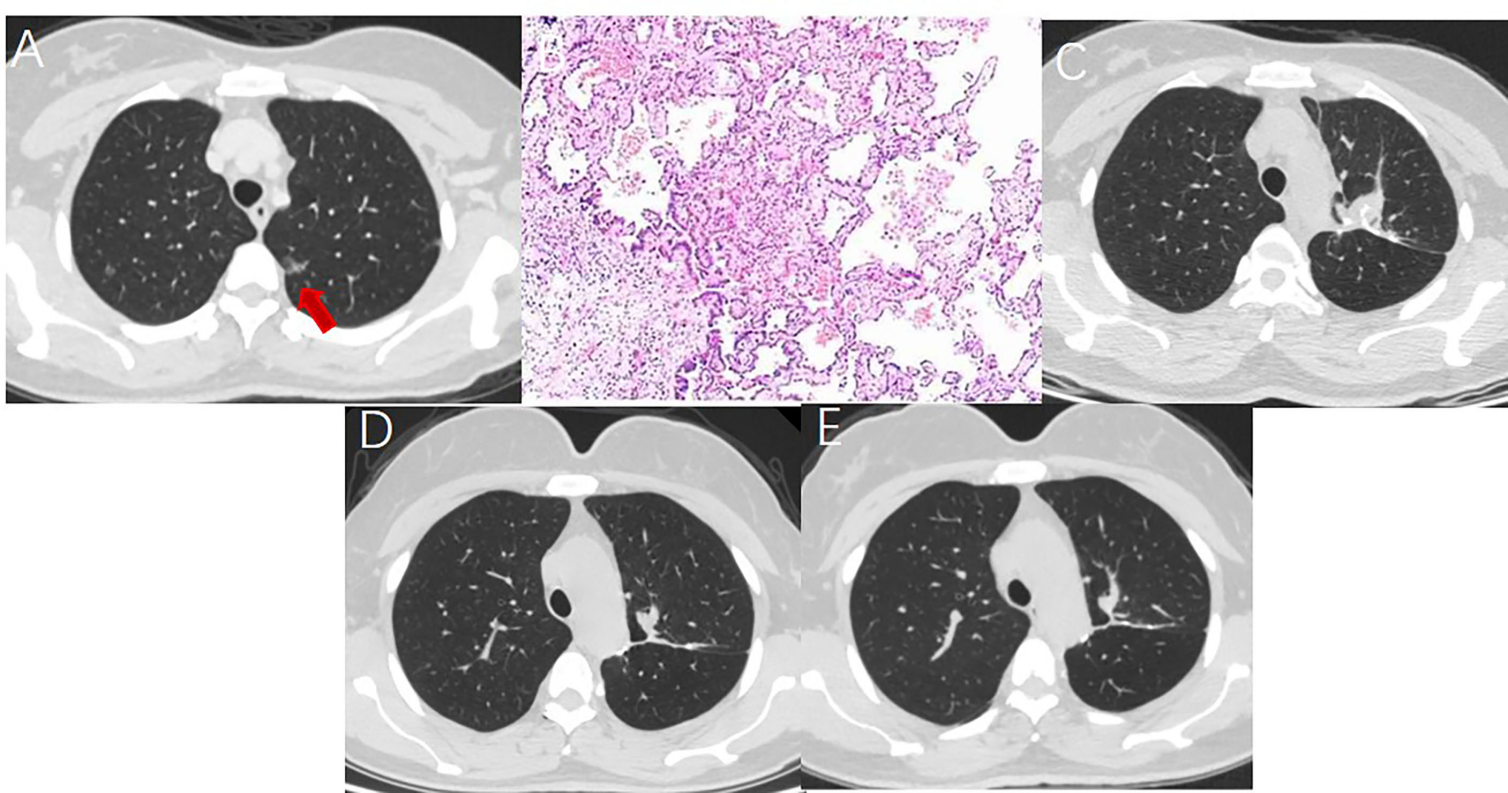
A 52 year-old woman with multiple GGNs underwent resection of apical posterior segment of left upper lobe and lymph node sampling. (A) A ground glass nodule with a diameter of 1.5cm located in the left upper lobe. (B) Postoperative pathology showed minimal invasive adenocarcinoma with no lymph node metastasis. (C) Soft tissue-density mass and linear stapler can be seen in the operation area on the CT image one month after surgery. (D) The soft tissue density mass in the operation area shrank 7 months after surgery. (E) The operation area formed fibrous scar 14 months after surgery.
